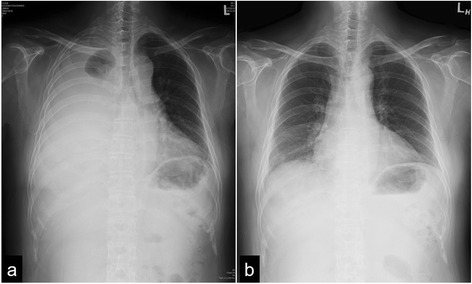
Chest X-ray films before indwelling drainage plus melphalan therapy (a, Feb. 14, 2017) and 2 months after completing six courses of melphalan treatment (b, Oct. 26, 2017)
